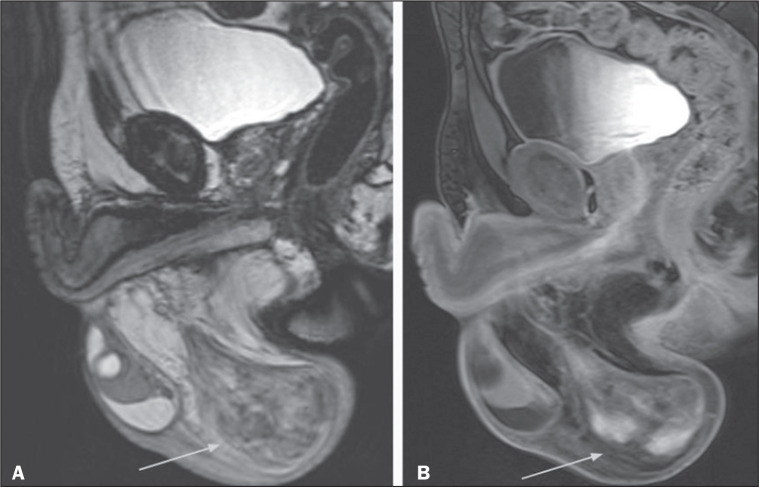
Liposarcoma of the scrotum. Sagittal T2-weighted image without fat
